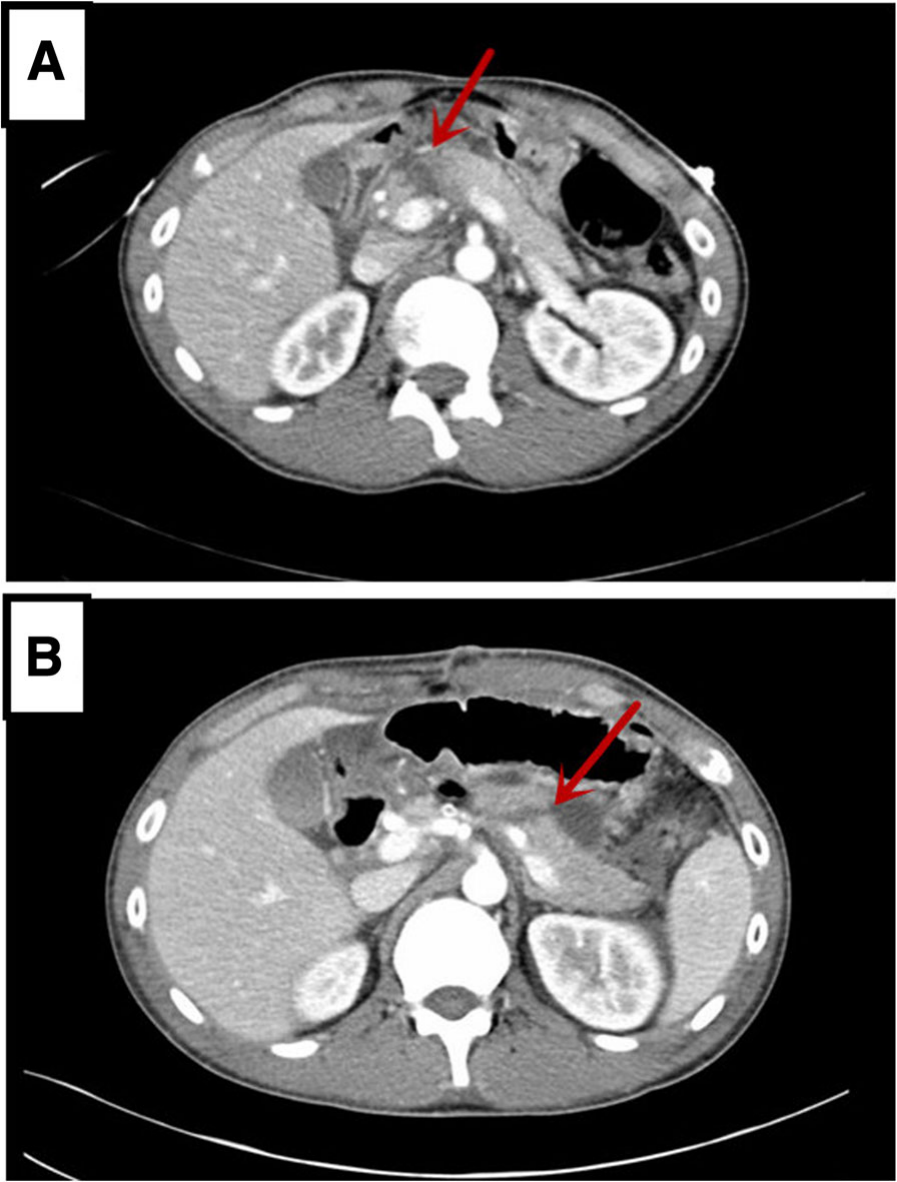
(a) Abdominal computed tomography (CT) reveal discontinuous pancreatic neck parenchyma, peripancreatic effusion, and hemorrhage (the red arrow indicates the pancreatic neck complete transection). (b) Postoperative abdominal CT show a small amount of gas and fluid in the abdominal cavity, and the anastomotic site of the pancreas and intestine healed well (the red arrow denotes the anastomotic site of the pancreas and intestine)
